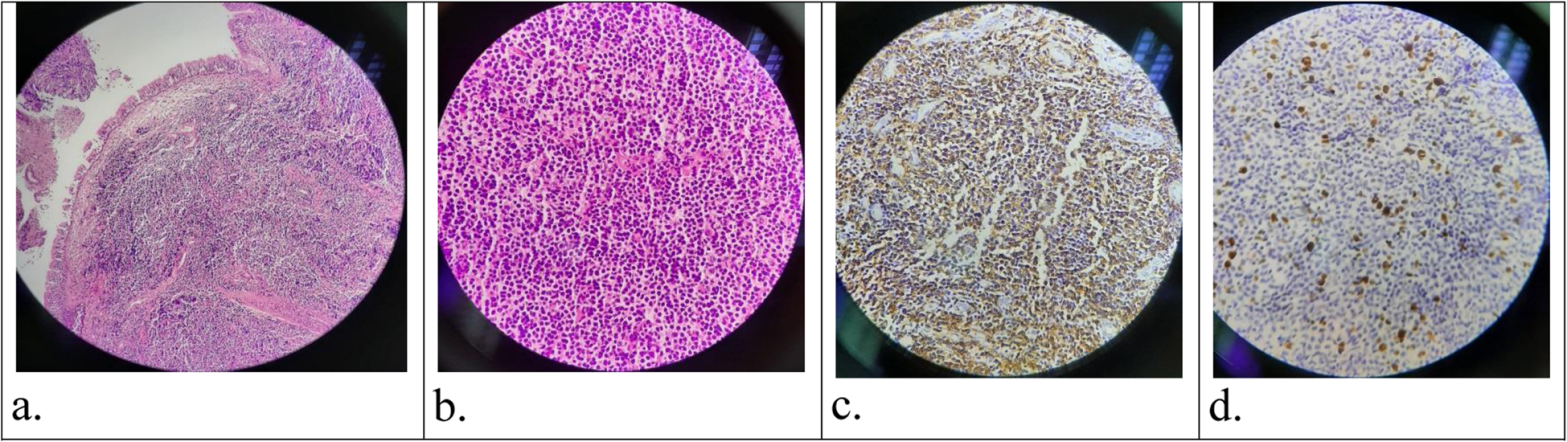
Coronal and sagittal planes of Pituitary and hypothalamus magnetic resonance imaging ± Gadolinium after completing chemotherapy
